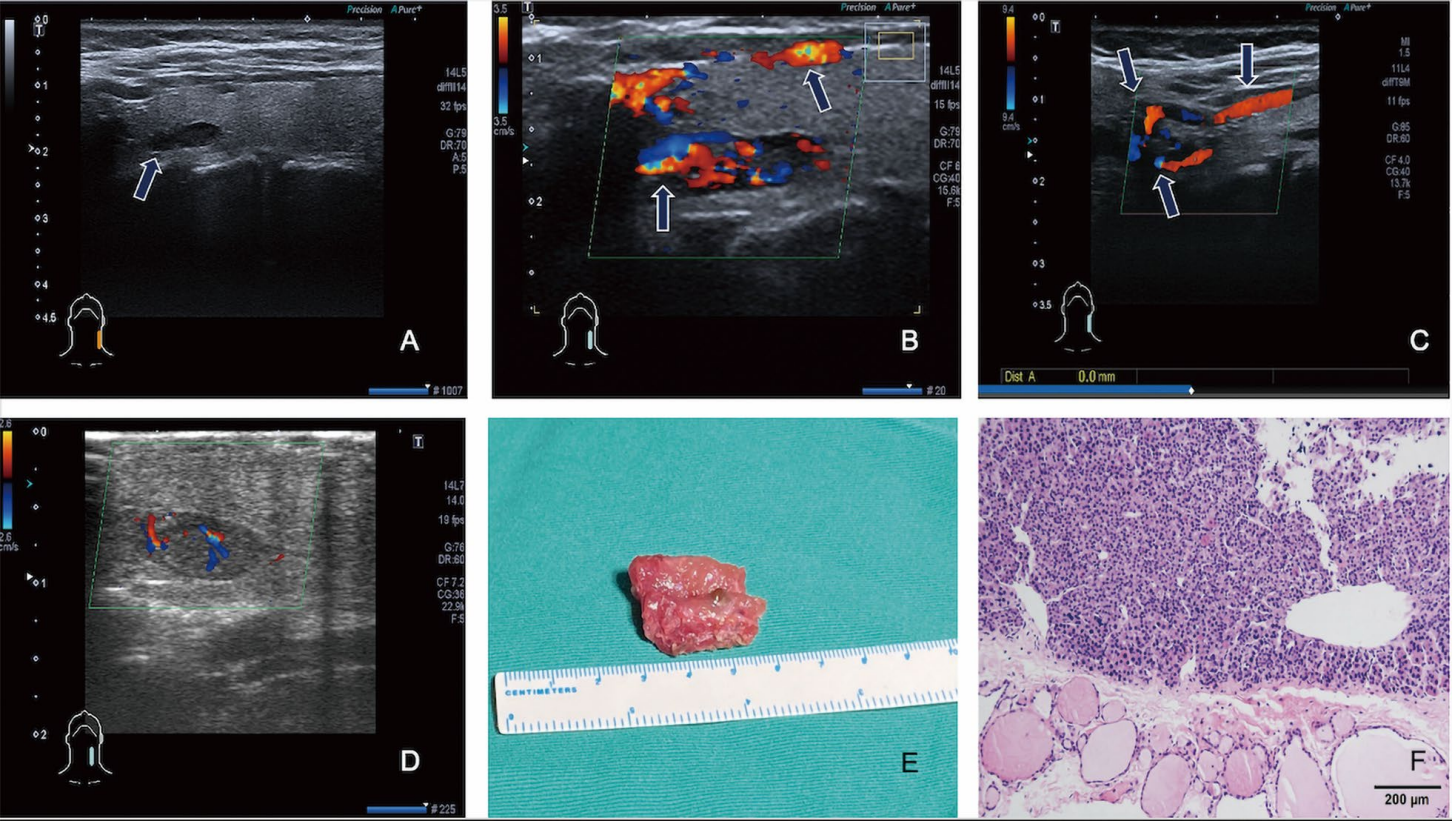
A complete type of intrathyroid parathyroid adenoma in the left lobe of thyroid. A homogeneously hypoechoic nodule in the left lobe of thyroid (a) that was negative in Tc-99m MIBI SPECT/CT, and the polar vascular sign (the supply vessel from the posterior branch of the superior thyroid artery) and anterior branch were detected by US (b, c). After clipping the superior thyroid artery intraoperatively, the blood supply in the nodule decreased significantly, which confirmed that the blood supply of the nodule came from the superior thyroid artery (d). After partial thyroidectomy and the nodule was resected, the US showed the parathyroid nodule was completely removed (e). The pathological results showed the parathyroid adenoma and surrounding thyroid tissue (f)
